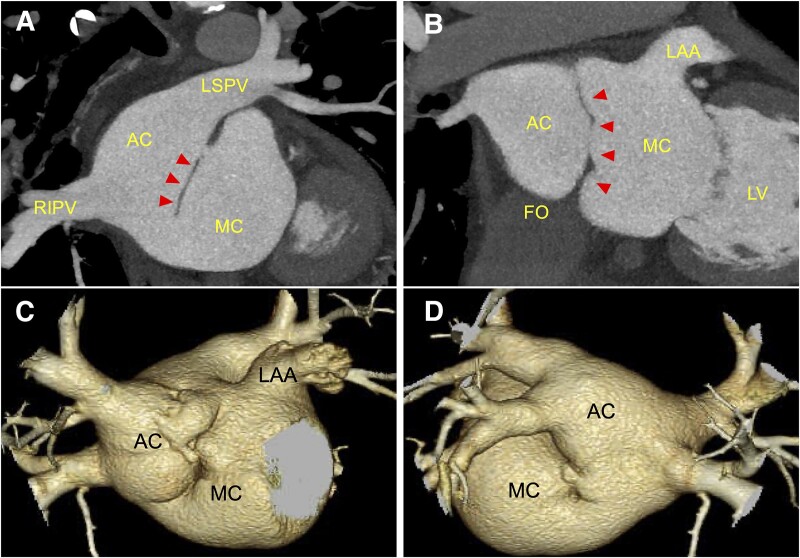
Multidetector computed tomography findings. (*A* and *B*) Coronal sections. The fossa ovalis is in contact with the accessory chamber. (*C* and *D*) Three-dimensional reconstruction. All pulmonary veins flow into the accessory chamber. LAA, left atrial appendage; LV, left ventricle; FO, fossa ovalis; AC, accessory chamber; MC, main chamber; RIPV, right inferior pulmonary vein; LSPV, left superior pulmonary vein. Arrow heads, membrane.
